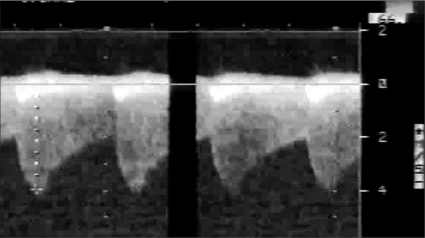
Continuous wave Doppler interrogation across the arch from suprasternal view after ductal closure showing systolic gradient 64 mm Hg, with diastolic spilling (continuous flow). Tr arch-transverse arch, Dao-descending aorta
